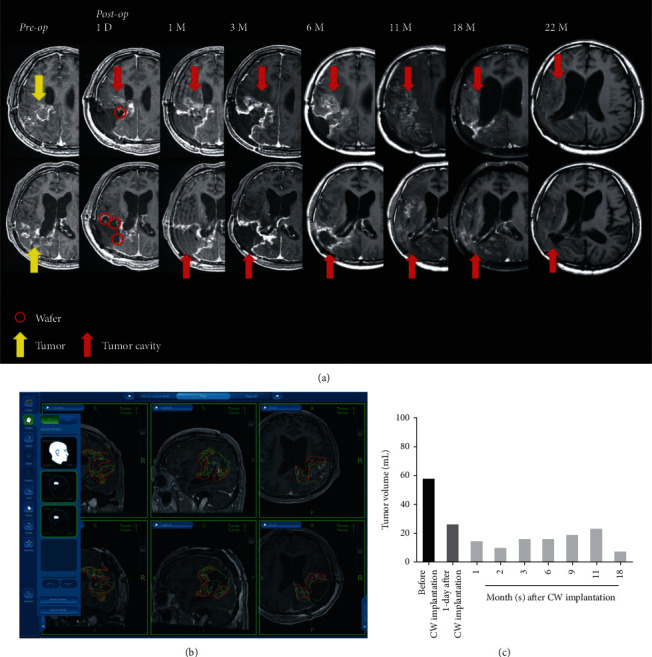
Clinical local control after intracranial implantation of Cerebraca Wafers, medication with TMZ, and infusion of pembrolizumab and CIK cells. (a) Axial MRI (T1-weighted with gadolinium enhancement) of the right parietal brain highlighting the cavity of tumor resection where CWs covered suspected residual tumors (red circles indicated CWs and yellow arrows marked resected tumors). During follow-up at 22 months with MRI, these enhancements were stable or had decreased without multifocal GBM (red arrows labeled tumor cavity). (b) Tumor volumes were calculated using regions of interest (ROI) in green line that correspond to pre-operative tumors and the red lines indicate residual malignant parts. (c) Extent of resection was ∼55% at day 1 of post-procedure, and the tumor volumes gradually regressed during 22 months after CW implantation.
